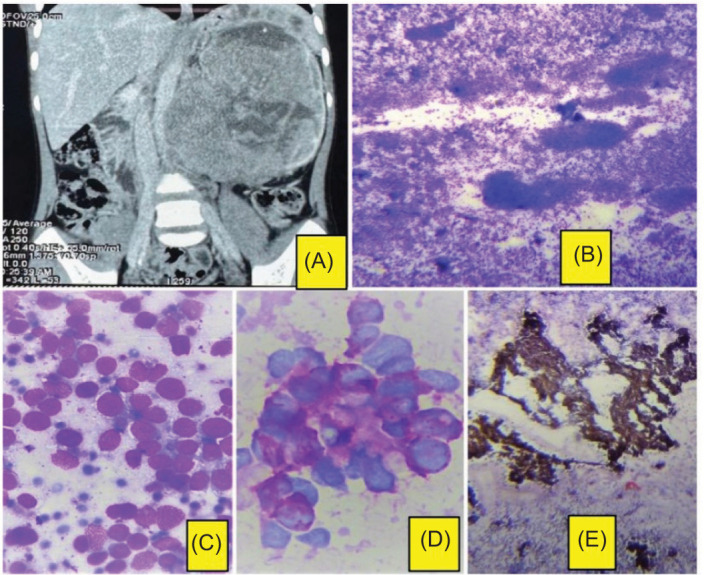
(A) CT Triphasic angiography shows large, ill-defined heterogeneously enhancing mass arising from the left kidney. (B–E) FNAC-highly cellular smears of atypical cells (×40). Dual population of atypical cells; cytoplasmic vacuolation in large cells (×400). Areas of calcification seen (×100). PAS positive tumor cells seen (×400).
